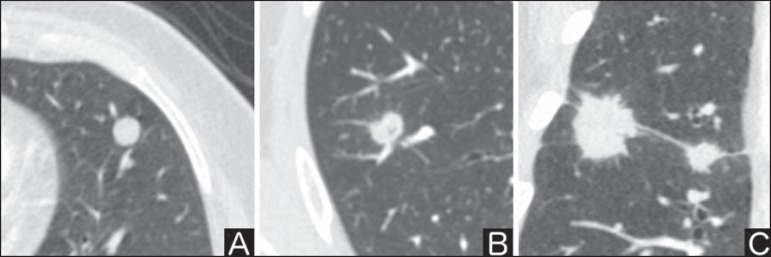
Margins.* Chest CT - lung window. A:
